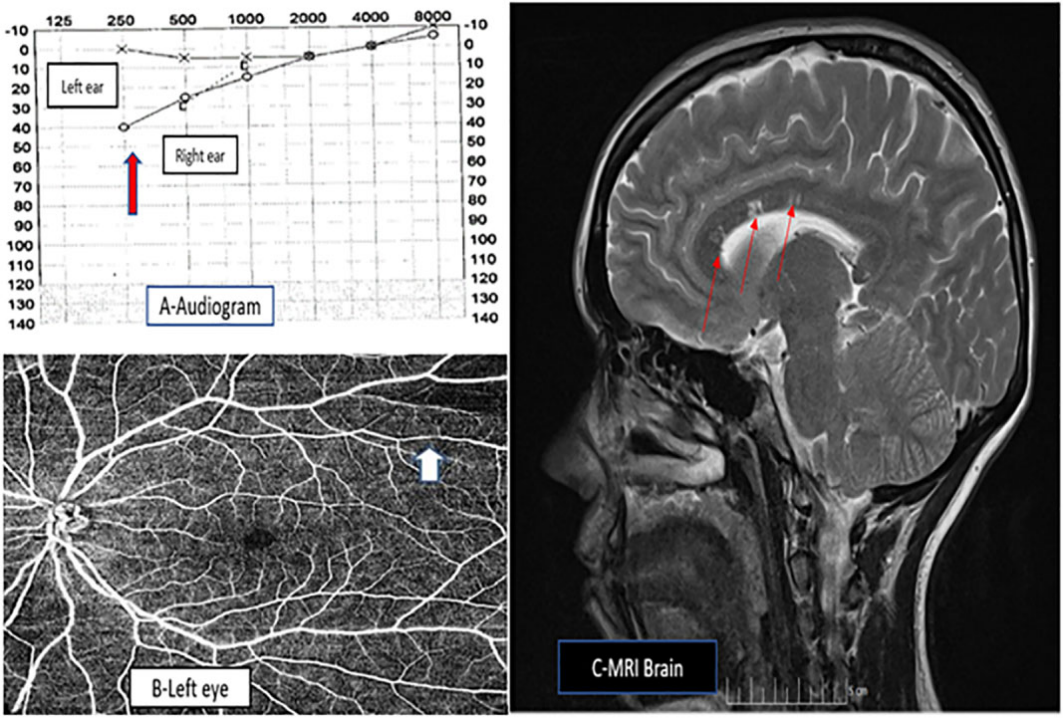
Susac syndrome (A) Audiogram showing sensorineural hearing loss at low frequency in right ear. (B) Optical Coherence Tomography angiogram showing superotemporal branch retinal artery occlusion in the left eye. (C) MRI brain sagittal view showing snowball lesions on T2 images.
